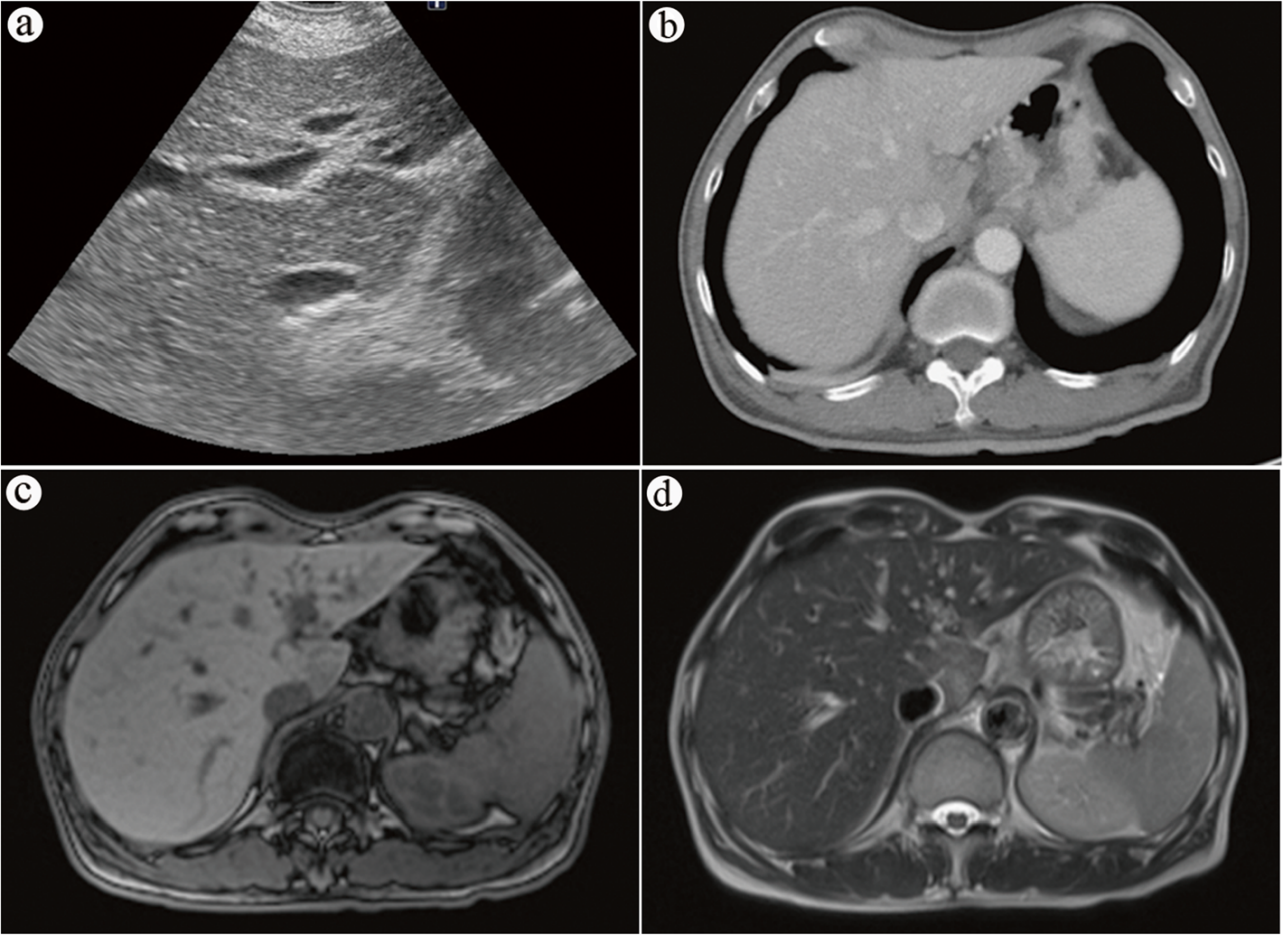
a Sonography of the liver shows hypoechoic appearance over caudate lobe. b Contrast-enhanced computed tomography shows focally less enhancement in S1 of the liver. c Magnetic resonance imaging (MRI) of the liver shows a low signal intensity over the caudate lobe in T1-weighted images. d MRI of the liver shows a high signal intensity over the caudate lobe in T2-weighted images
